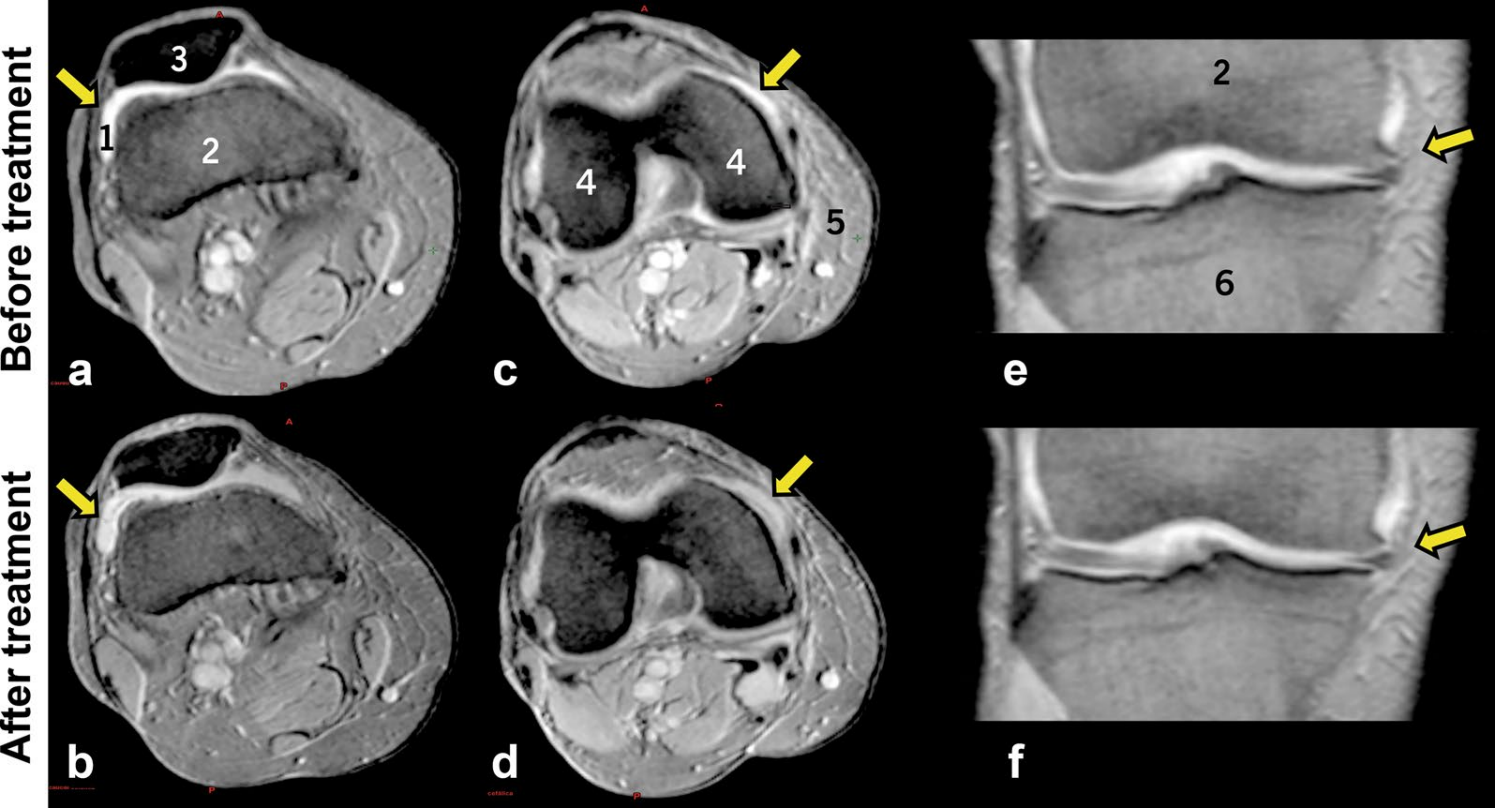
Nuclear magnetic resonance images of the knee joint before and after treatment with BIOF2. Axial views at the height of the patella (a, b) and the femoral condyles (c, d) and coronal views (e, f), before and after 12 months of treatment, respectively. The arrows indicate the zones in which treatment generated a beneficial change, with respect to cartilage thickness or continuity. 1: cartilage, 2: femur, 3: patella 4: femoral condyle, 5: muscle, 6: tibia
